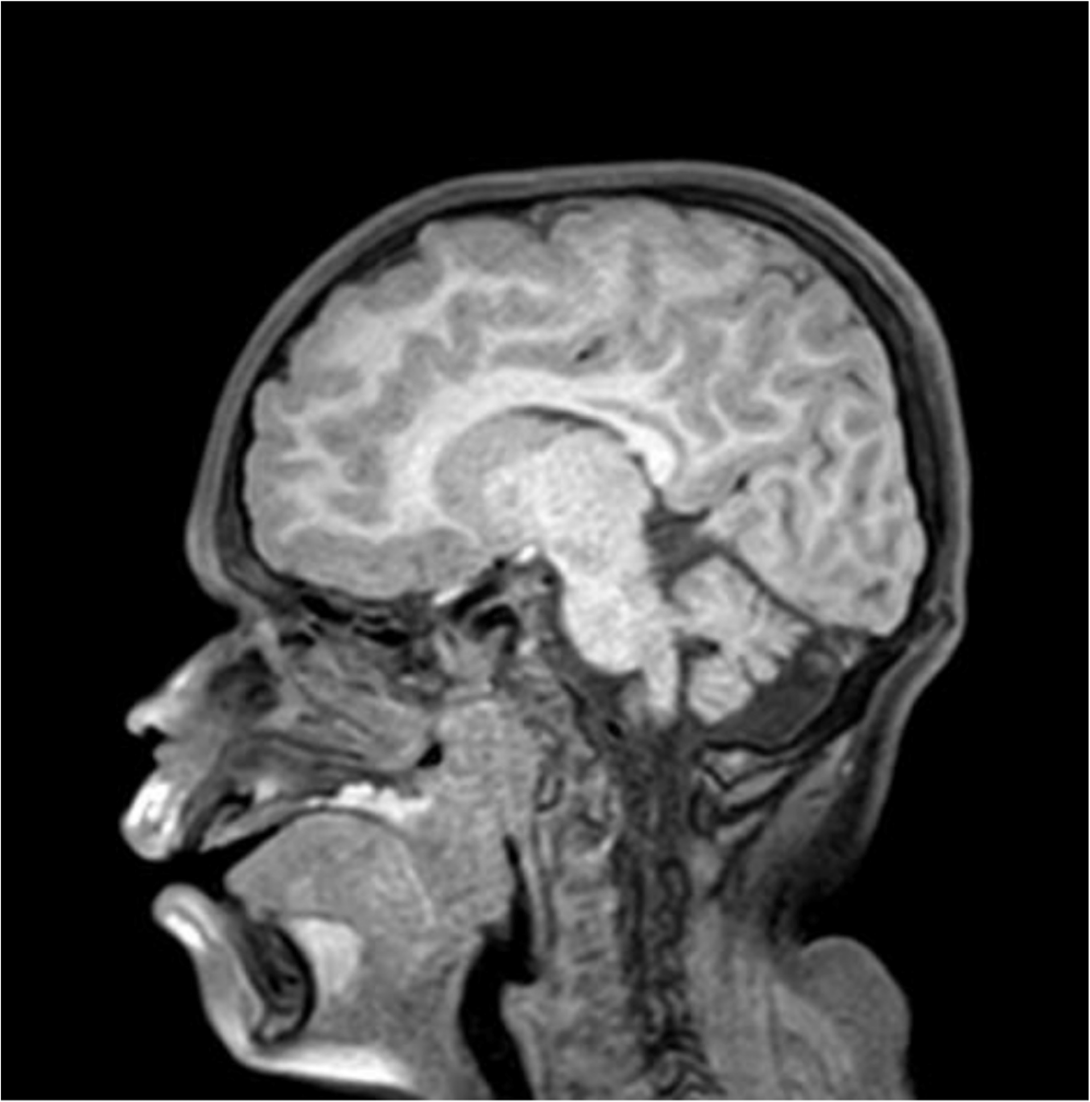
MRI scan showing a very narrow air column in the larynx and pharynx space along with the significant adenotonsillay hypertrophy
